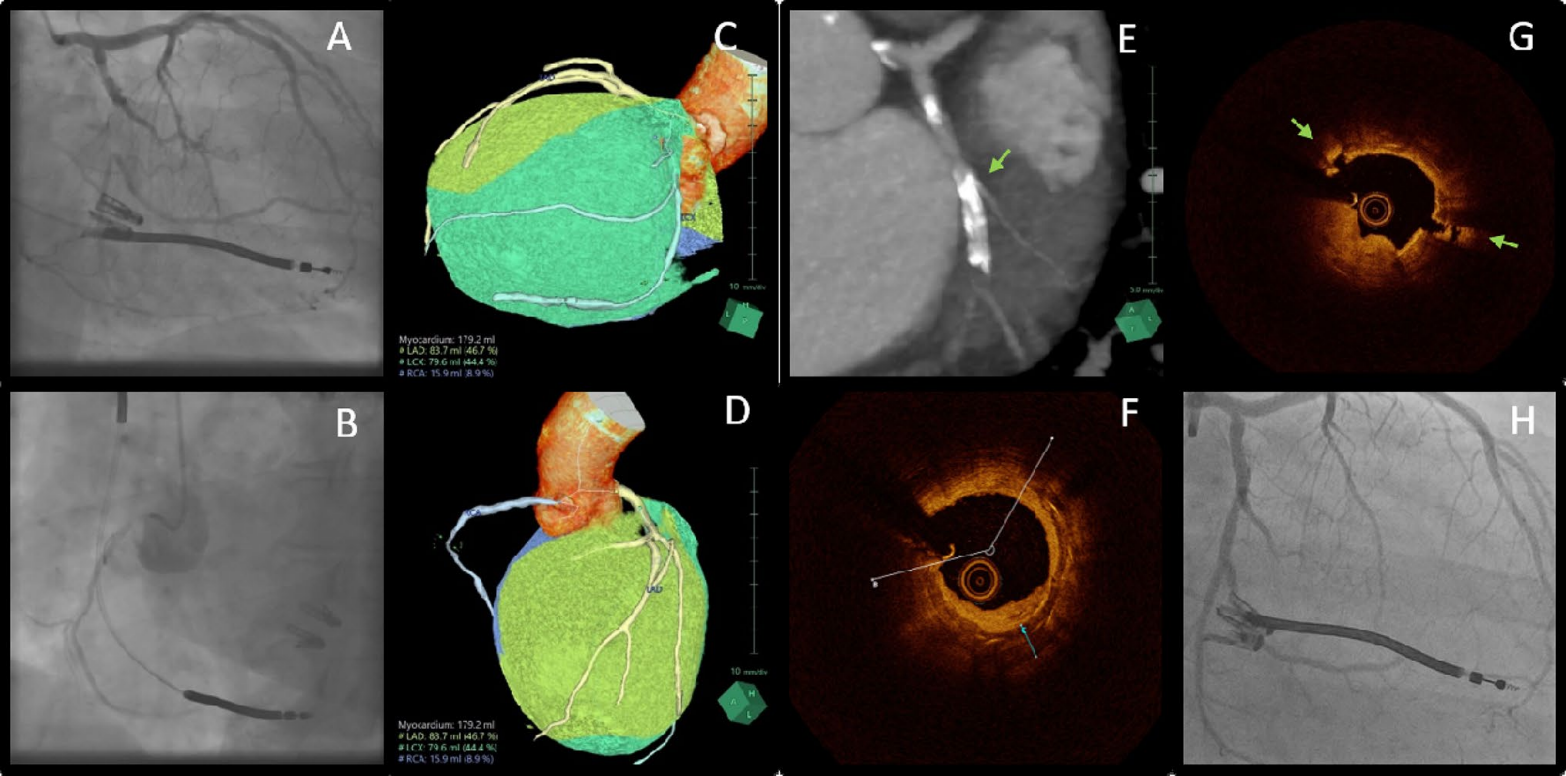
A Angiography shows CTO of the mid LCx. B Angiography shows the ostial RCA CTO with antegrade collateral filling. C, D CT shows a 44.4% supply of the myocardial volume by the LCx and a 8.9% supply by the RCA. E CT (Slab-MIP) shows the CTO in the mid LCx with heavy calcification in the proximal part of the CTO segment. (Green Arrow). F OCT shows deep calcification with an angle of 250 degrees and thickness of 0.70 mm. G OCT shows superficial calcification with cracks (Green arrows) after cutting balloon dilatation and intact deep calcification. H Final angiography of the LCx after stents implantation
